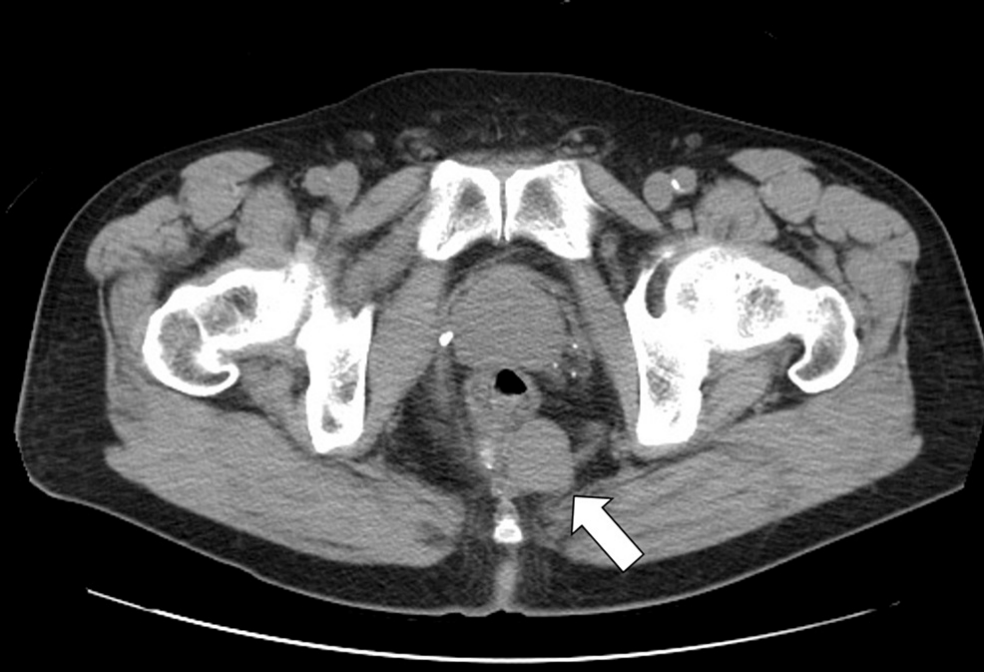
Abdominal CT findings CT incidentally revealed a well-defined multilocular mass (3 cm in diameter) on the dorsal surface of the rectum (arrow).
